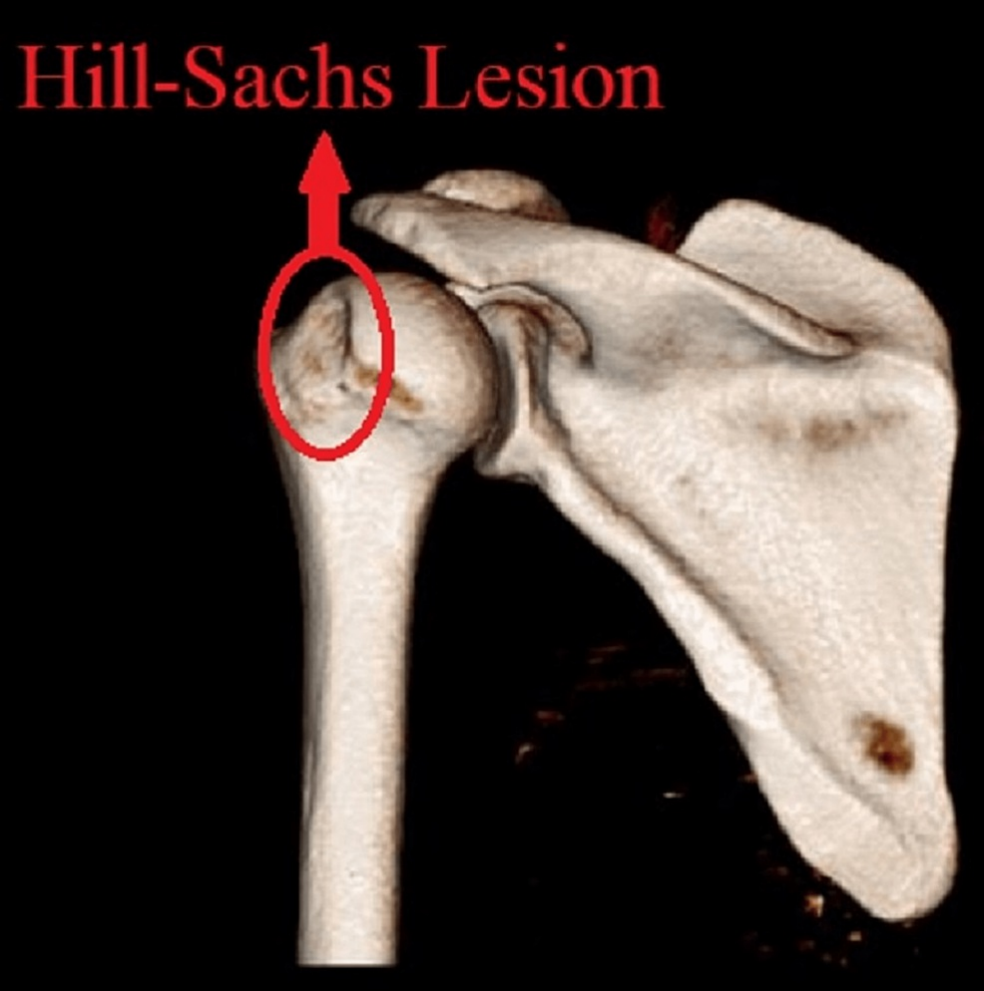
A 3D CT scan of the left shoulder joint
